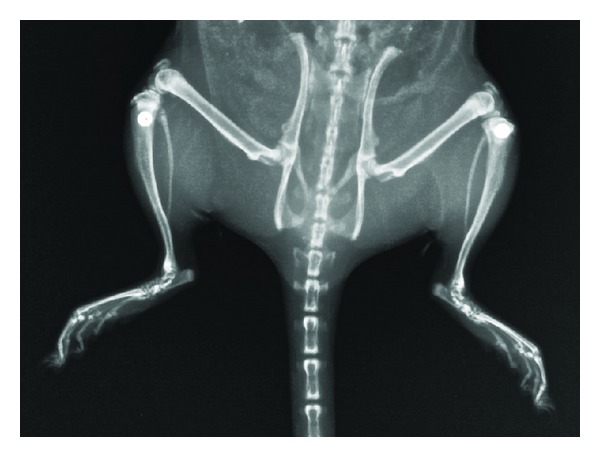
X-ray examination after surgery with the implant properly positioned in the proximal tibial metaphysis.
